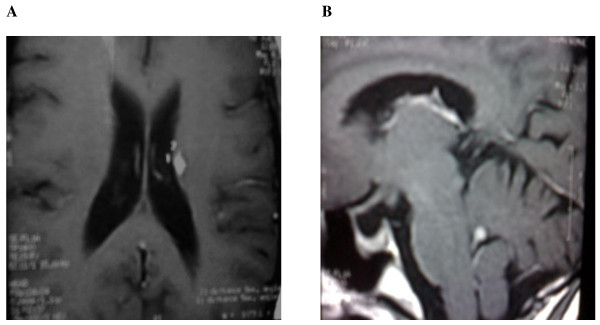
Four months later after steroid treatment, MRI of the brain showing new process developed in the CNS.
